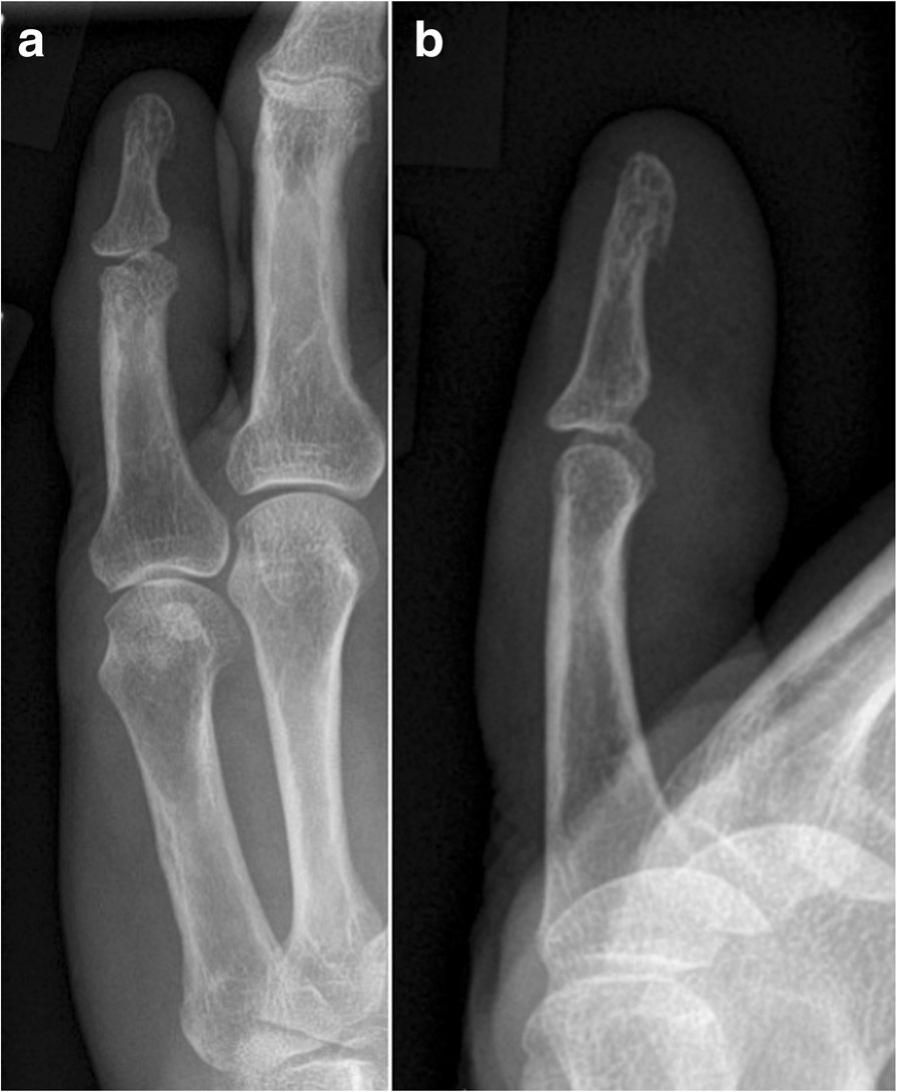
Radiograph of patient 2 showing (a) posteroanterior and (b) lateral views of the small finger
